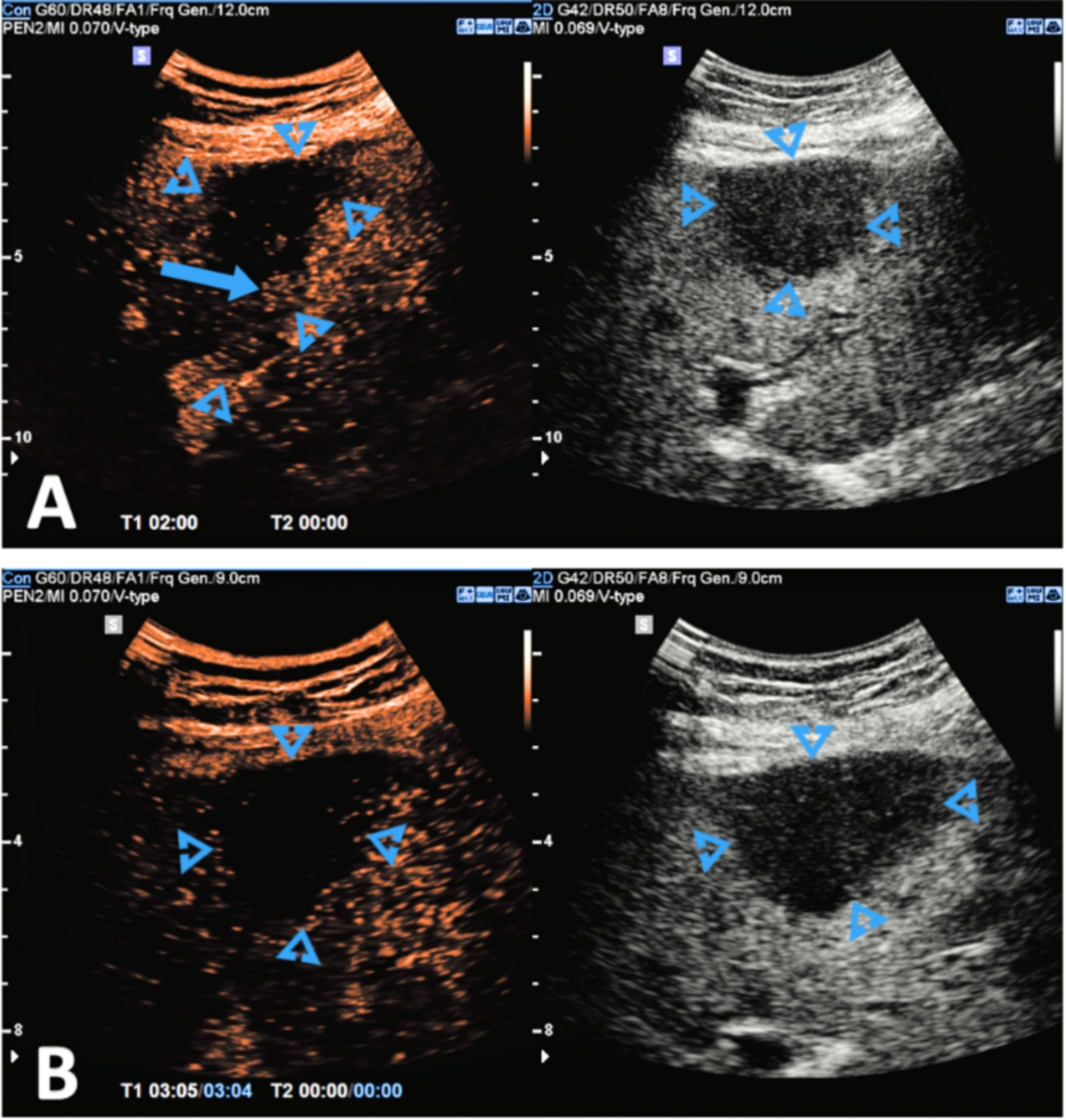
(A-B) After administration of IV An ultrasound contrast agent (SonoVue). The largest lesion is monitored throughout the arterial phase. The mass again shows internal vascularity with slightly delayed enhancement, followed by washout in the portal. Image Courtesy: Dr Balint Boltz, Radiopaedia.org, ID:70877; https://radiopaedia.org/cases/70877/studies/81075?lang=us IV, intravenous
